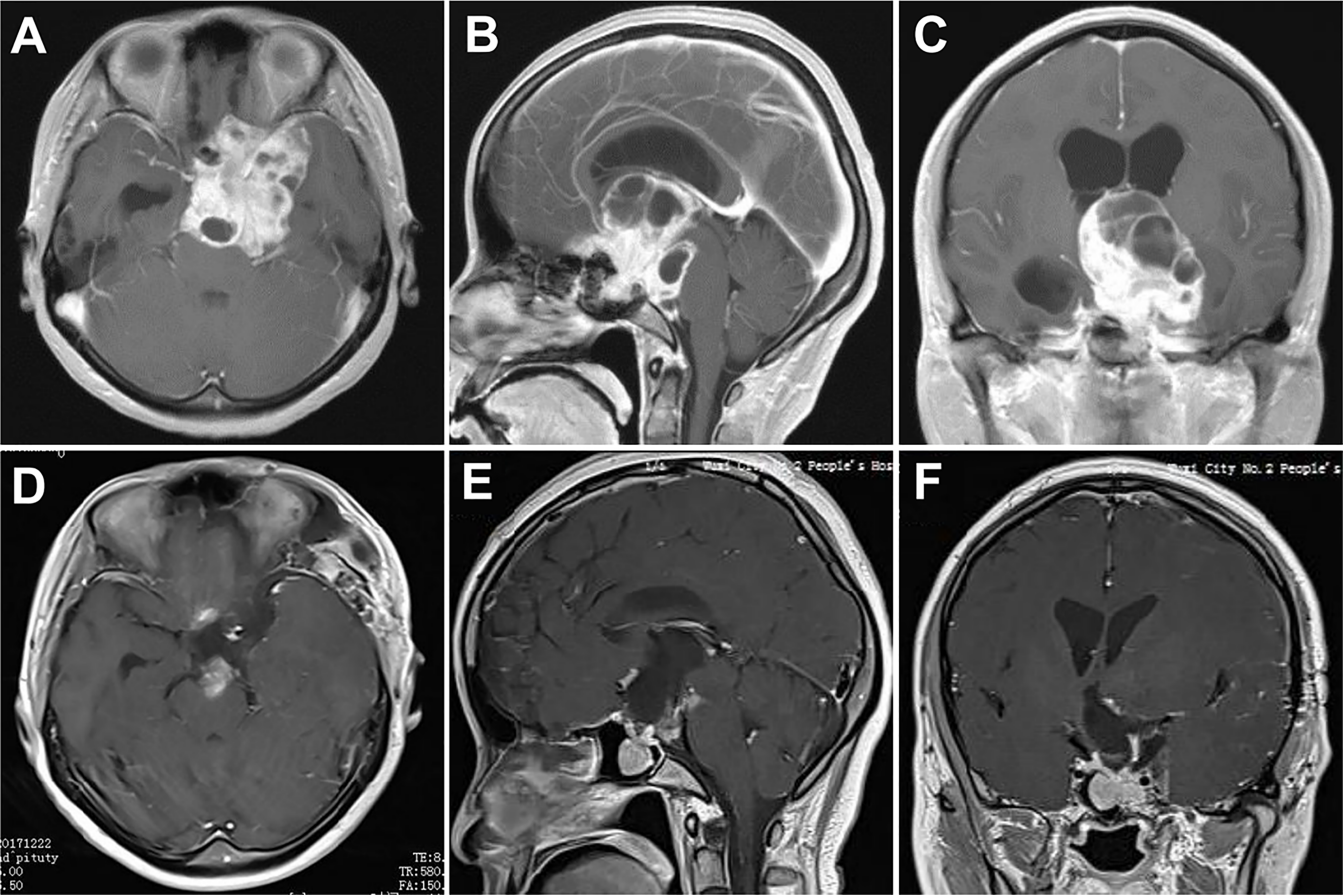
Case 3. Pre- and postoperative imaging findings. (A–C) Preoperative axial (A), sagittal (B) and coronal (C) gadolinium-enhanced T1-weighted MRI images showing a giant pituitary adenoma with extensive suprasellar extension. The tumor fills the entire third ventricle and invades into the sphenoid sinus. (D–F) Postoperative axial (D), sagittal (E) and coronal (F) gadolinium-enhanced T1-weighted MRI images showing near total resection of the pituitary tumor with residue in the sphenoid sinus.
